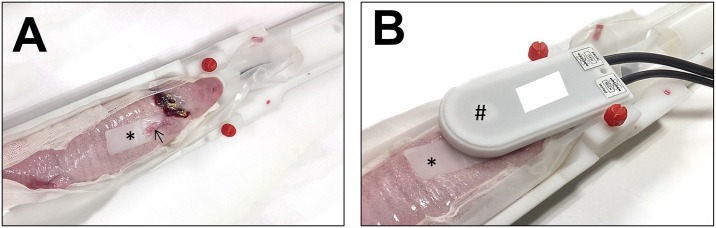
MR image acquisition. A: The anesthetized axolotl lies in left decubitus position in the trench with its right upper arm (arrow) resting in neutral position and flattened on a plastic plate (asterisk). B: A radiofrequency-receive surface coil (indicated as #) was placed on the top of the right upper arm.
